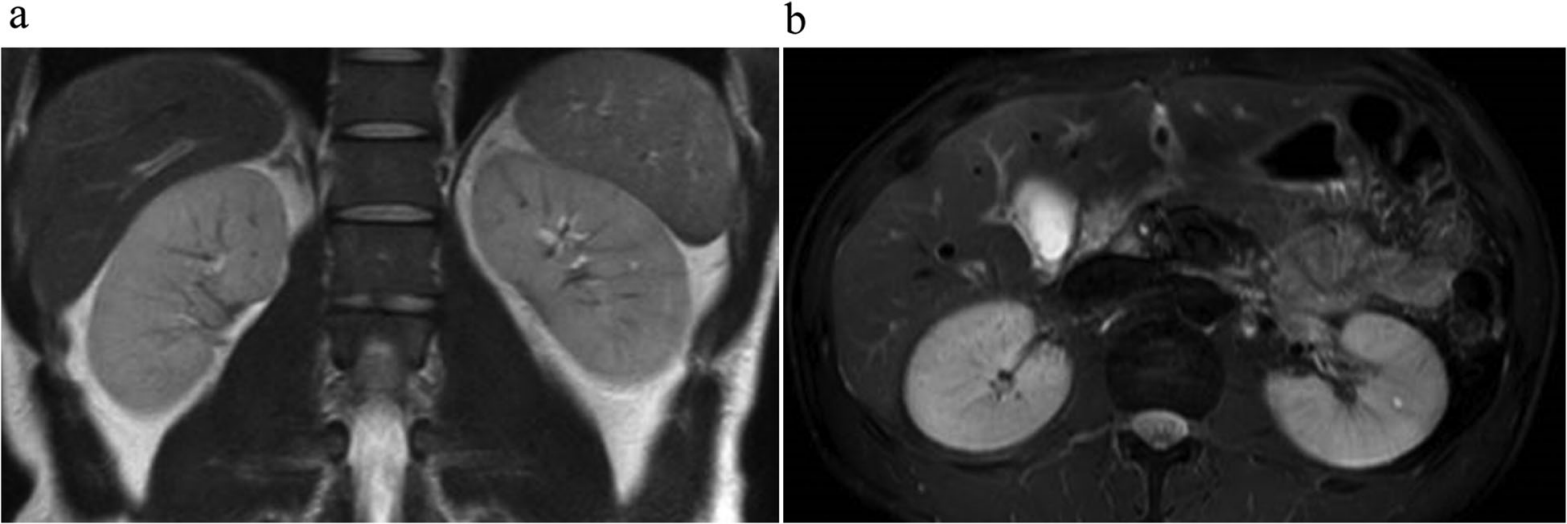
Renal MRI image. Cuneiform low-signal region found in the T2-weighted renal MRI image, indicating heterogeneous ischemia. a Coronal, b Axial. MRI, magnetic resonance imaging
